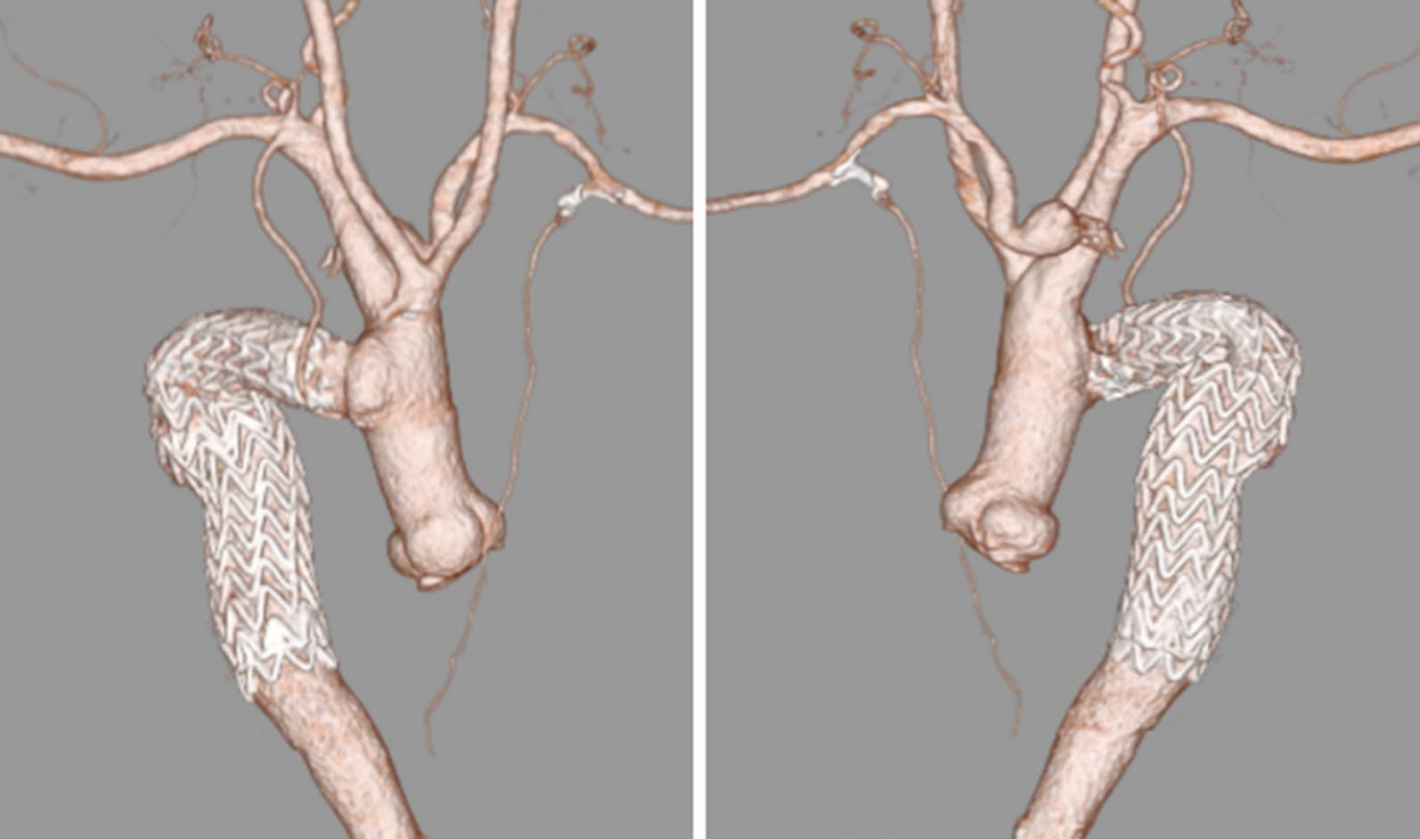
Postoperative computed tomography with 3D reconstruction. Anterior (a) and posterior (b) views show no contrast medium extravasation
